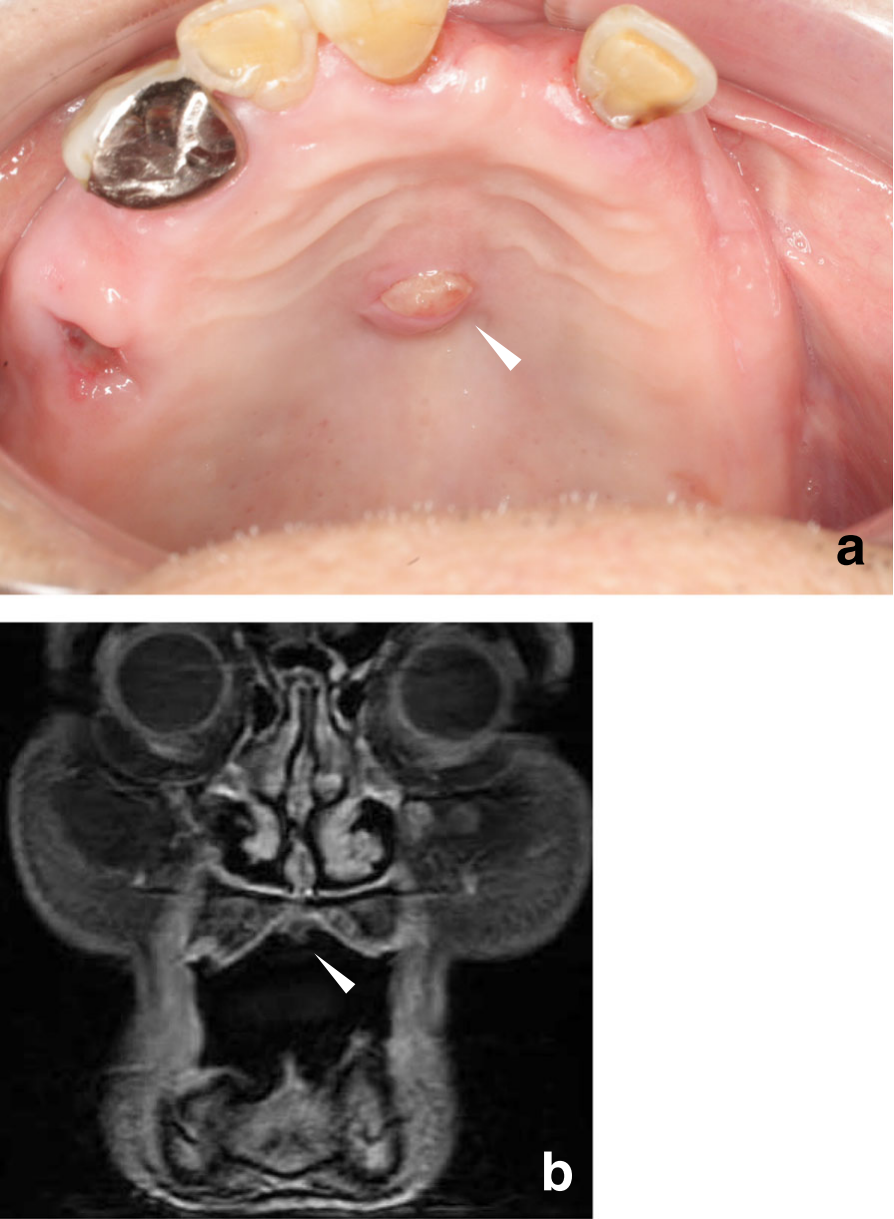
Clinical findings of the mass of the palate mucosa. a The arrowhead showed a mass of the palate mucosa. It was a small (major axis; 7 mm) and round mass with smooth surface (arrowhead). b Magnetic Resonance Imaging (MRI) showed a small mass localized in the palate mucosa (arrowhead)
